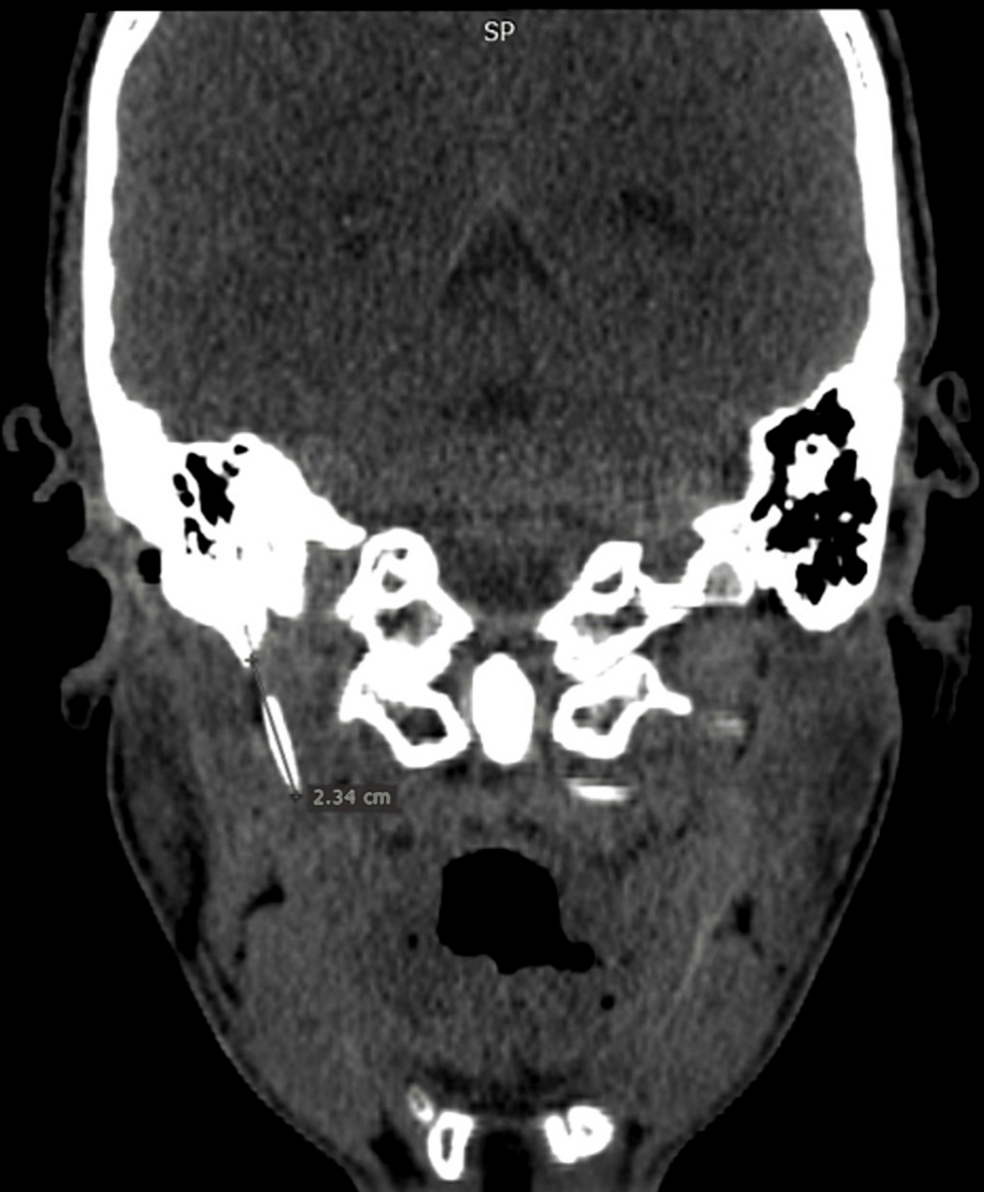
Coronal CT showing length of right styloid process within normal range
